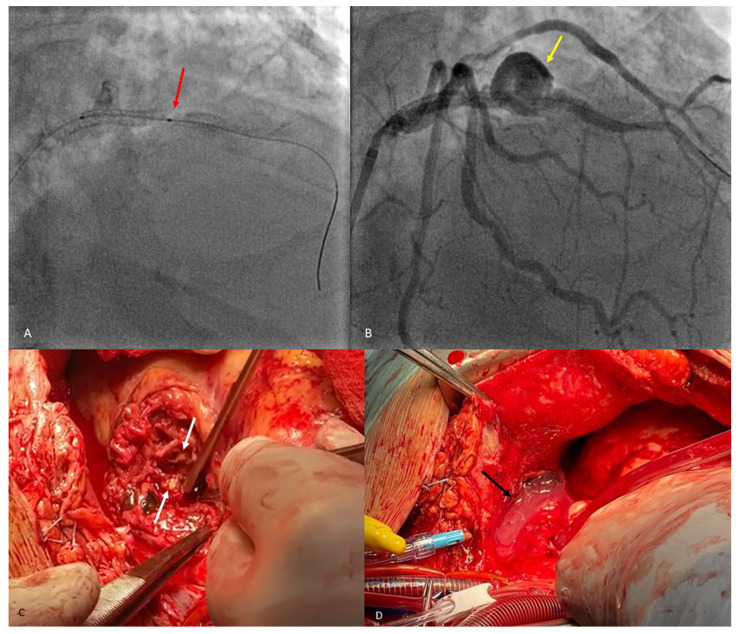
A classic coronary angiography was performed next, where a type IV distal stent fracture involving the proximal LAD stent with dehiscence and slight migration ((A), red arrow) associated with a coronary pseudoaneurysm ((B), yellow arrow) were observed. An attempt was made to install a pharmacologically active post-dilation balloon stent in order to treat the pseudoaneurysm, but without success, which is why the patient was transferred to the cardiovascular surgery ward. The surgical procedure was performed under general anesthesia with orotracheal intubation and extracorporeal circulation, and the final diagnosis was a stent fracture associated with pseudoaneurysm formation and infected pericardial hematoma. Intraoperative view of the complete stent fracture and separation ((C), white arrows). Infected pericardial hematoma ((D), black arrow). The case is atypical, as the stent fracture was type IV (complete transverse fracture of the stent with separation into two fragments and displacement) and occurred at the level of the anterior descending artery. The most common stent fractures reported in the literature involve the right coronary artery, with a more tortuous course, and type III and IV fractures have the lowest incidences [4]. Coronary pseudoaneurysms following stenting are rare complications with an incidence of 0.3–6% and usually arise approximately 6–9 months after the intervention, but cases have been reported earlier than 2 months after the procedure [5]. Unlike similar reports in the literature [6], the attempt to implant a new drug-eluting stent in our patient failed. Finally, surgical revascularization proved to be the appropriate treatment strategy. After removing the pseudoaneurysm and performing an aorto-coronary bypass, the patient showed a favorable recovery and was discharged in a stable condition. Invasive coronary angiography is considered the gold standard for diagnosing intra-stent restenosis, although the invasive nature of the procedure comes with associated risks of mortality and morbidity. Computed tomography angiography (CTA) is a non-invasive imaging technique highly beneficial for follow-up consultations. Coronary artery intrastent restenosis detection has been determined to have high specificity when at least a 64-multislice CT technique is used. CTA can and should also be used when varying symptoms appear after an interventional coronary procedure [7].
